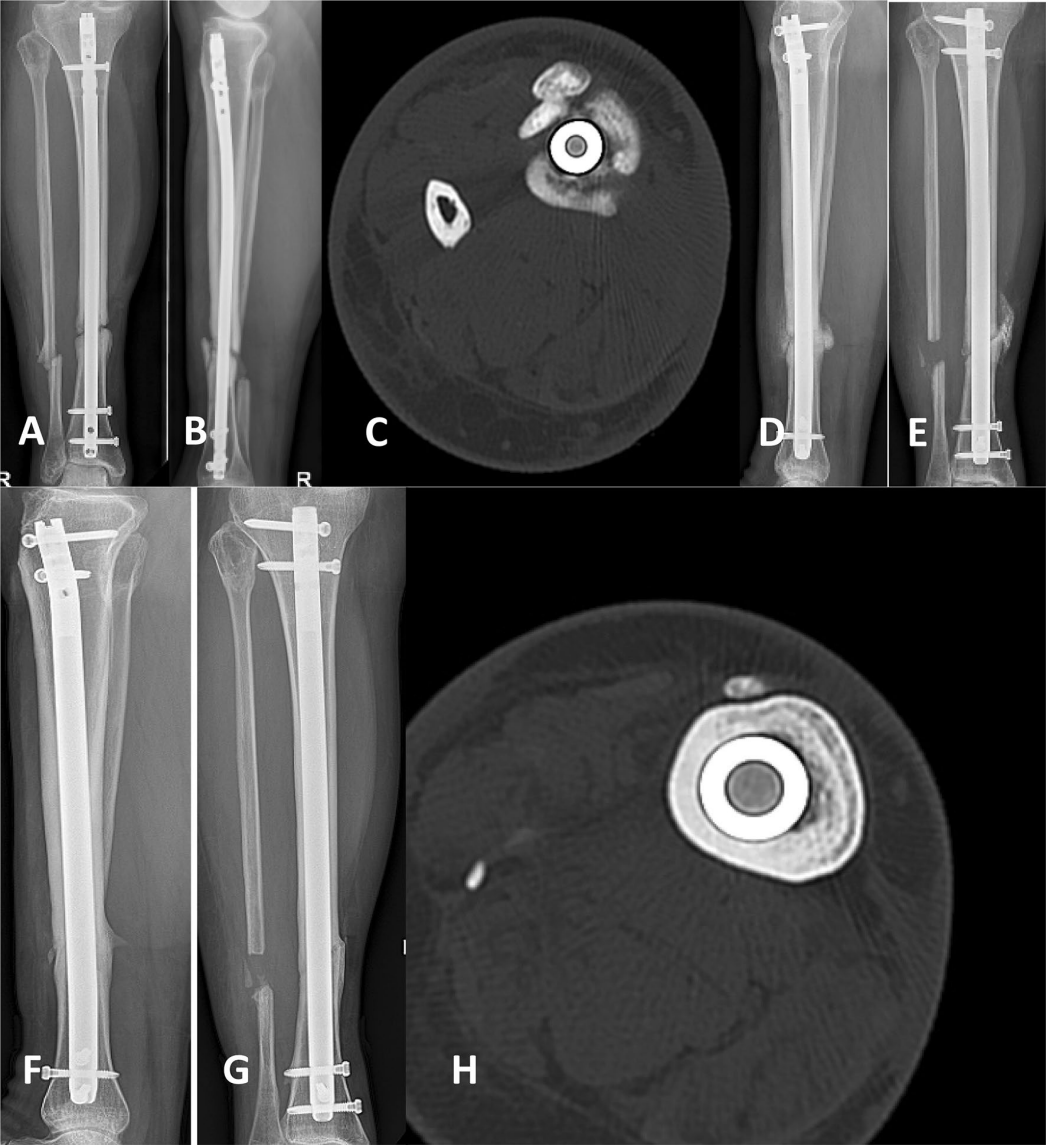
Late FRI 12 months after surgery with non-union of the tibial shaft after intramedullary nailing of a II° open tibial fracture (AO-42.A2). AP/lateral X-rays and axial CT scan showed non-union (a–c). After local debridement and intramedullary reaming, Cerament™ G was inserted, followed by internal stabilization with intramedullary nailing (d, e). After 18 months full bone consolidation was seen (f, g) as showed in AP/lateral X-rays and axial CT scan
